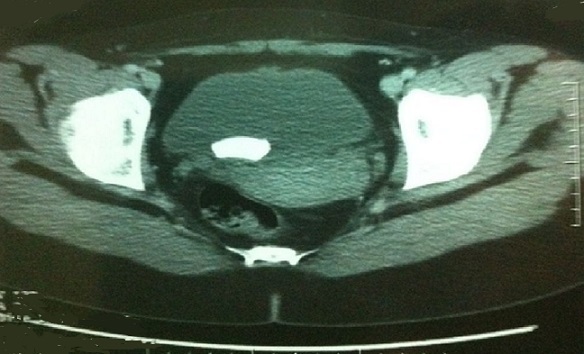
Pelvic computed tomography shows bladder stone (2 cm)
